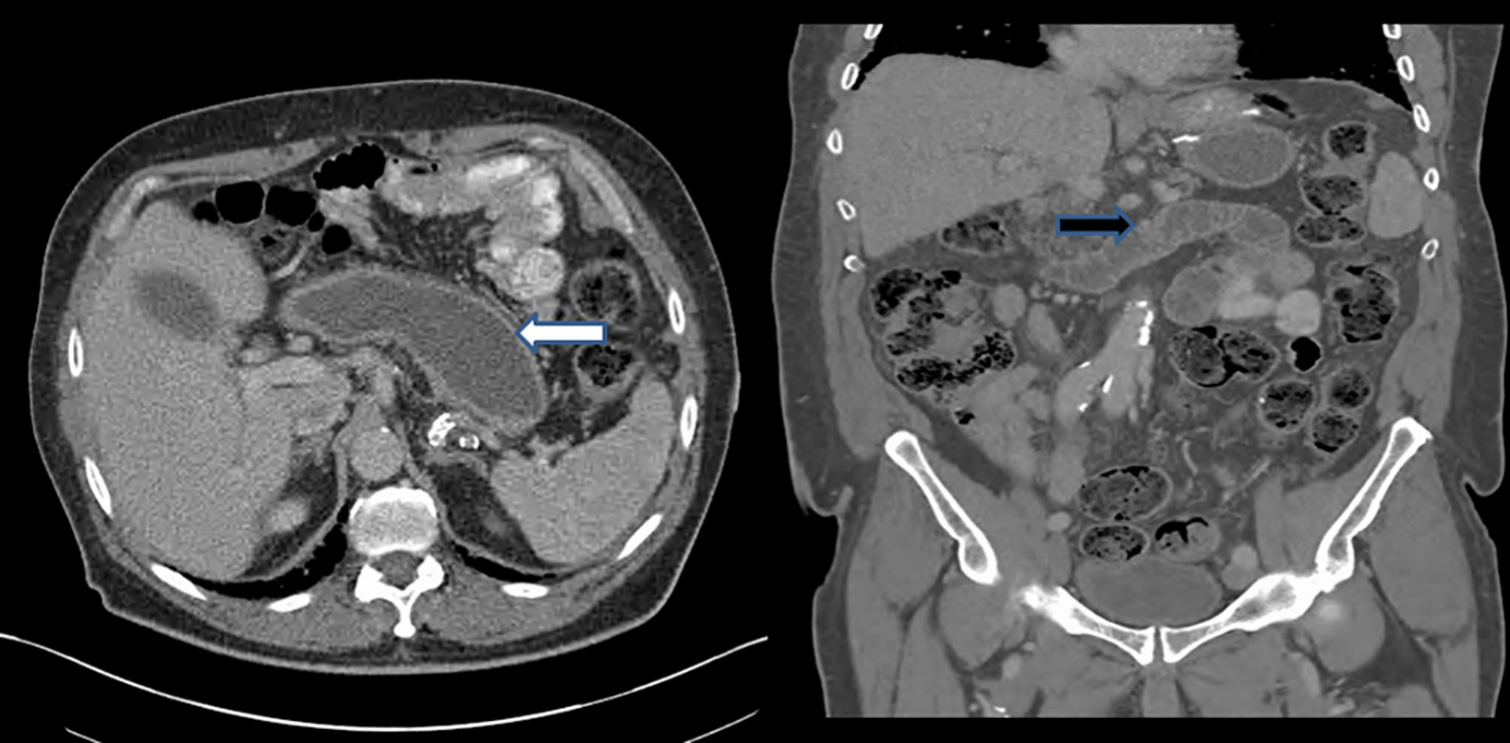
CT scan images show dilated gastric remnant (white arrow) and dilated biliopancreatic limb (black arrow).
